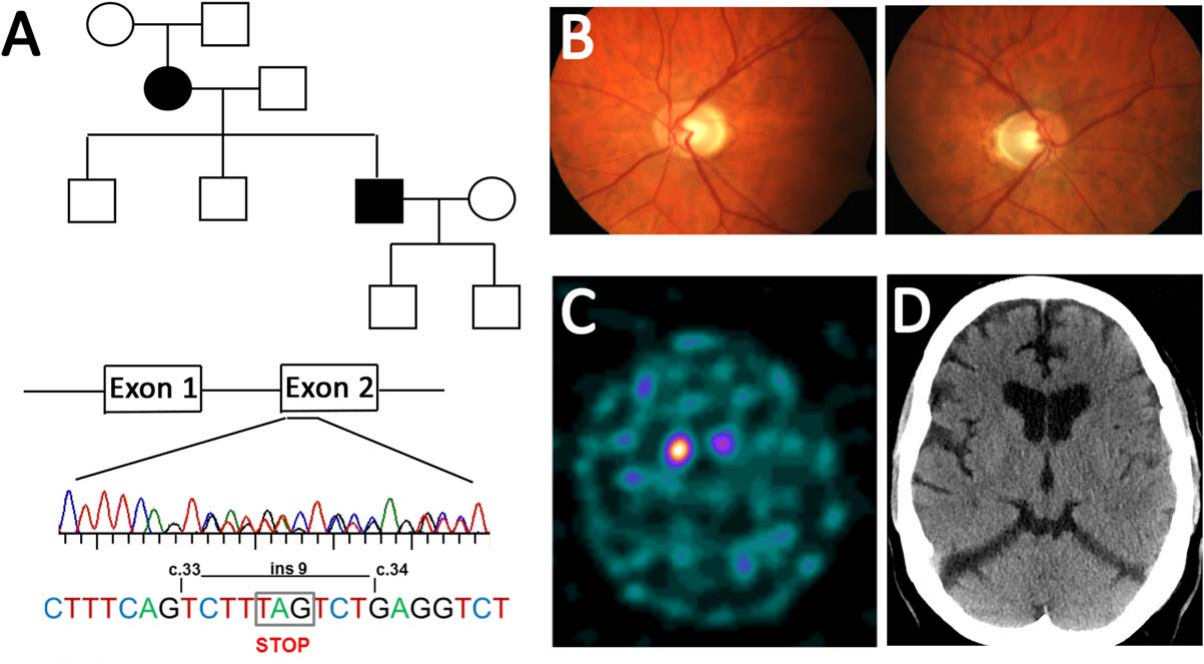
Clinical information. (A) Family tree of the index case who presented at the age of 46 with syndromic parkinsonism and his mother who manifested with pure optic atrophy until the age of 84. The OPA1 mutation consisted of a 9 base pair insertion introducing a stop codon in exon 2 leading to haploinsufficiency. (B) Bilateral optic atrophy in index case. (C) DaTScan showing evidence of asymmetric dopaminergic cell loss in the index case. (D) Recent CT brain of the index case showing mild generalised involutional changes without signal change within the basal ganglia. CT = computed tomography; OPA1 = optic atrophy 1. [Color figure can be viewed at http://www.annalsofneurology.org]
